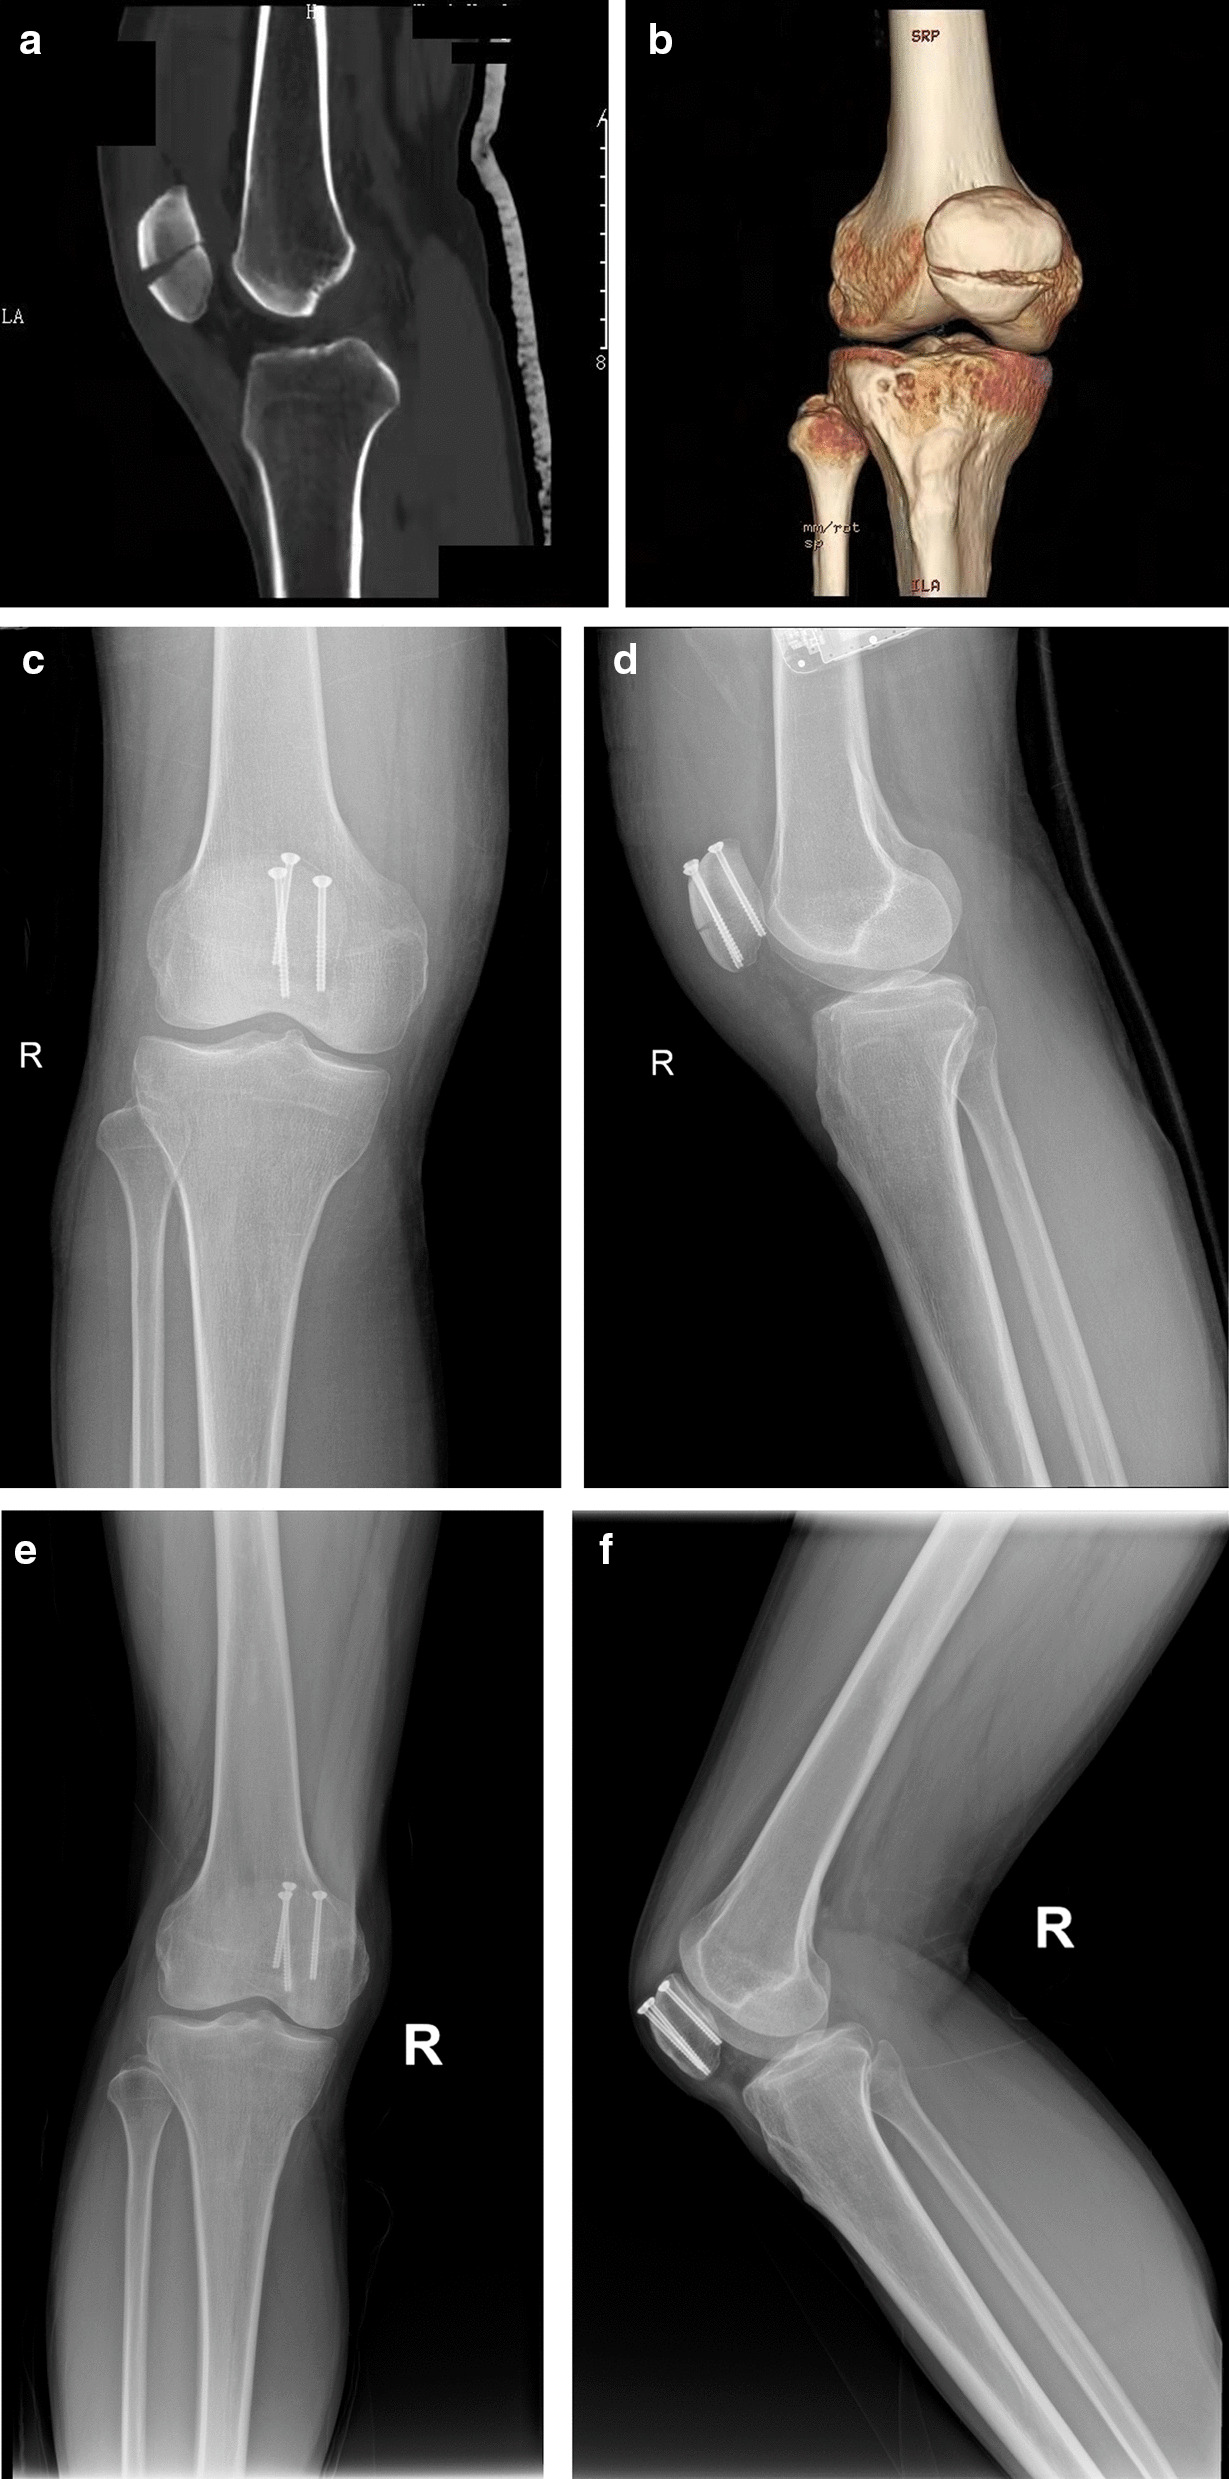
A full series of radiographs showing a typical case's condition before (A, B), after (C, D), and during the follow-up period of the surgery (E, F)
